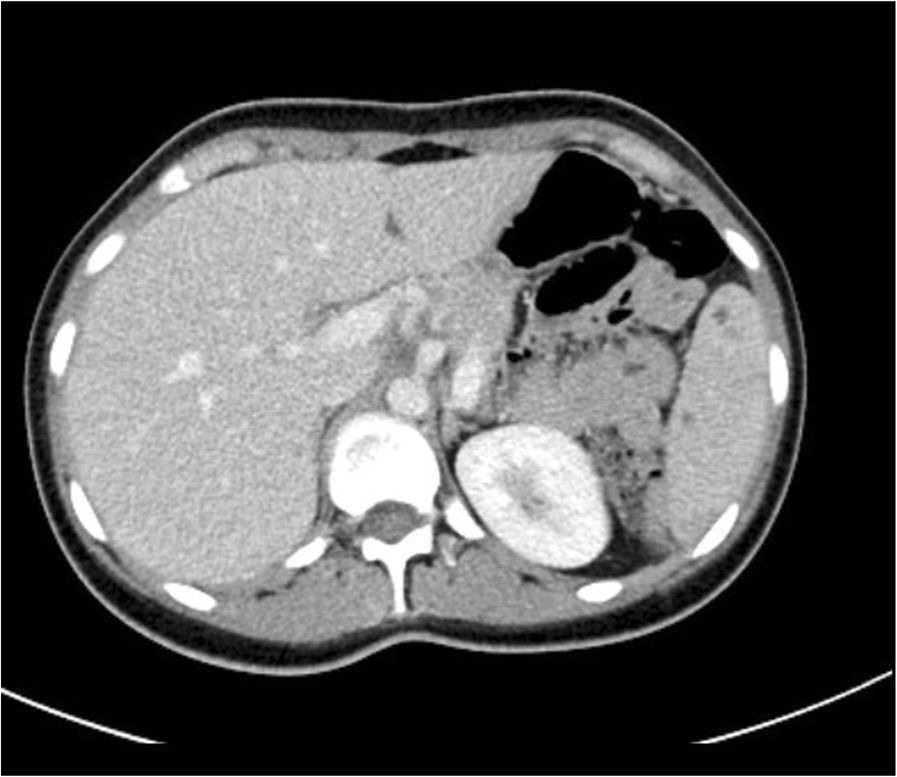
Abdominal CT scan. The CT scan shows multiple anechoic hypoechogenic nodules of the spleen, suggestive of abscesses or splenic lymphoma
